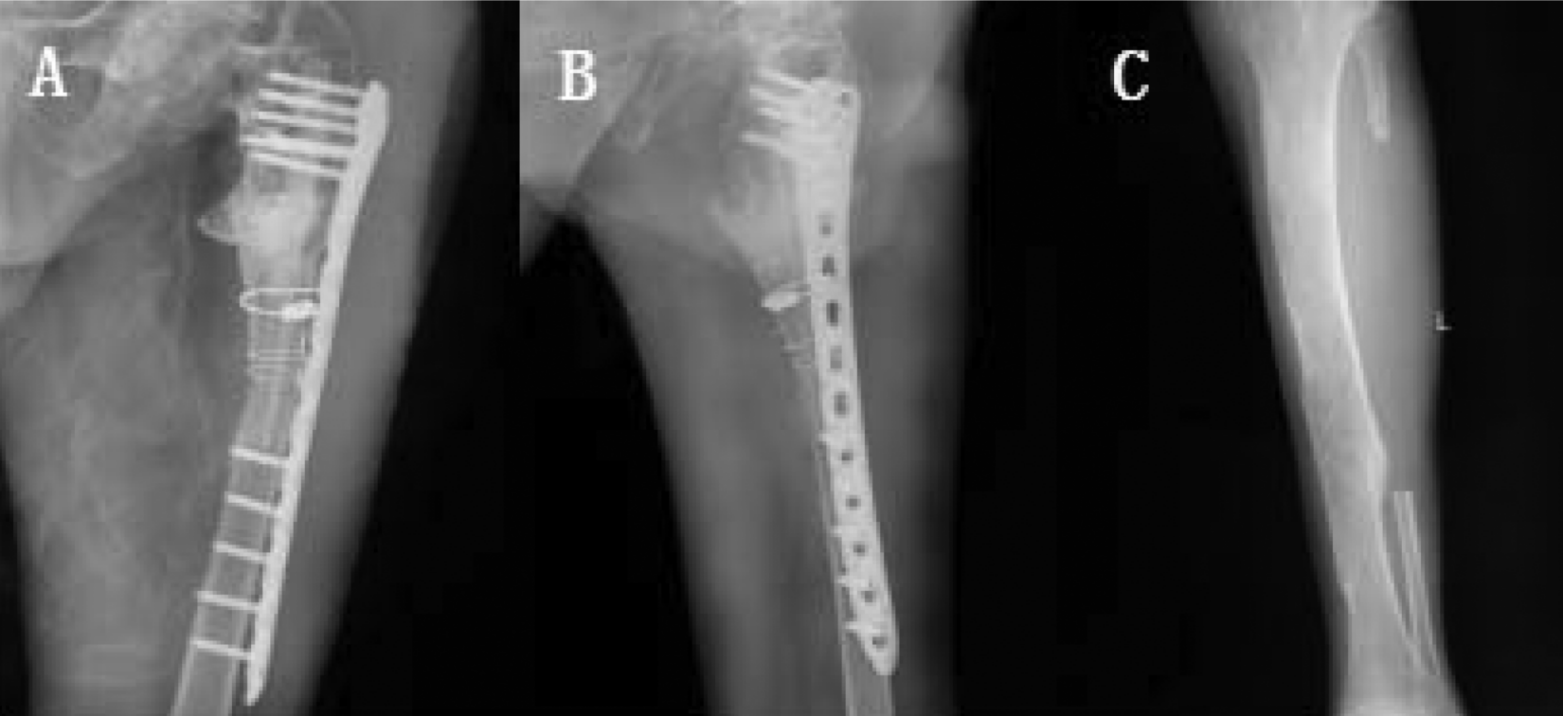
(A) Intraoperative fluoroscopy showing the fracture. Anatomically repositioned with internal fixation in good position. (B) A 12-month postoperative review image of front and side x-rays showing fracture healing, with fusion at the femoral pseudoarticulation and no loosening of the internal fixation. (C) A 12-month postoperative review of the left lower extremity with a 12-posterior x-ray of the fibula revealing no fracture of the tibia.
